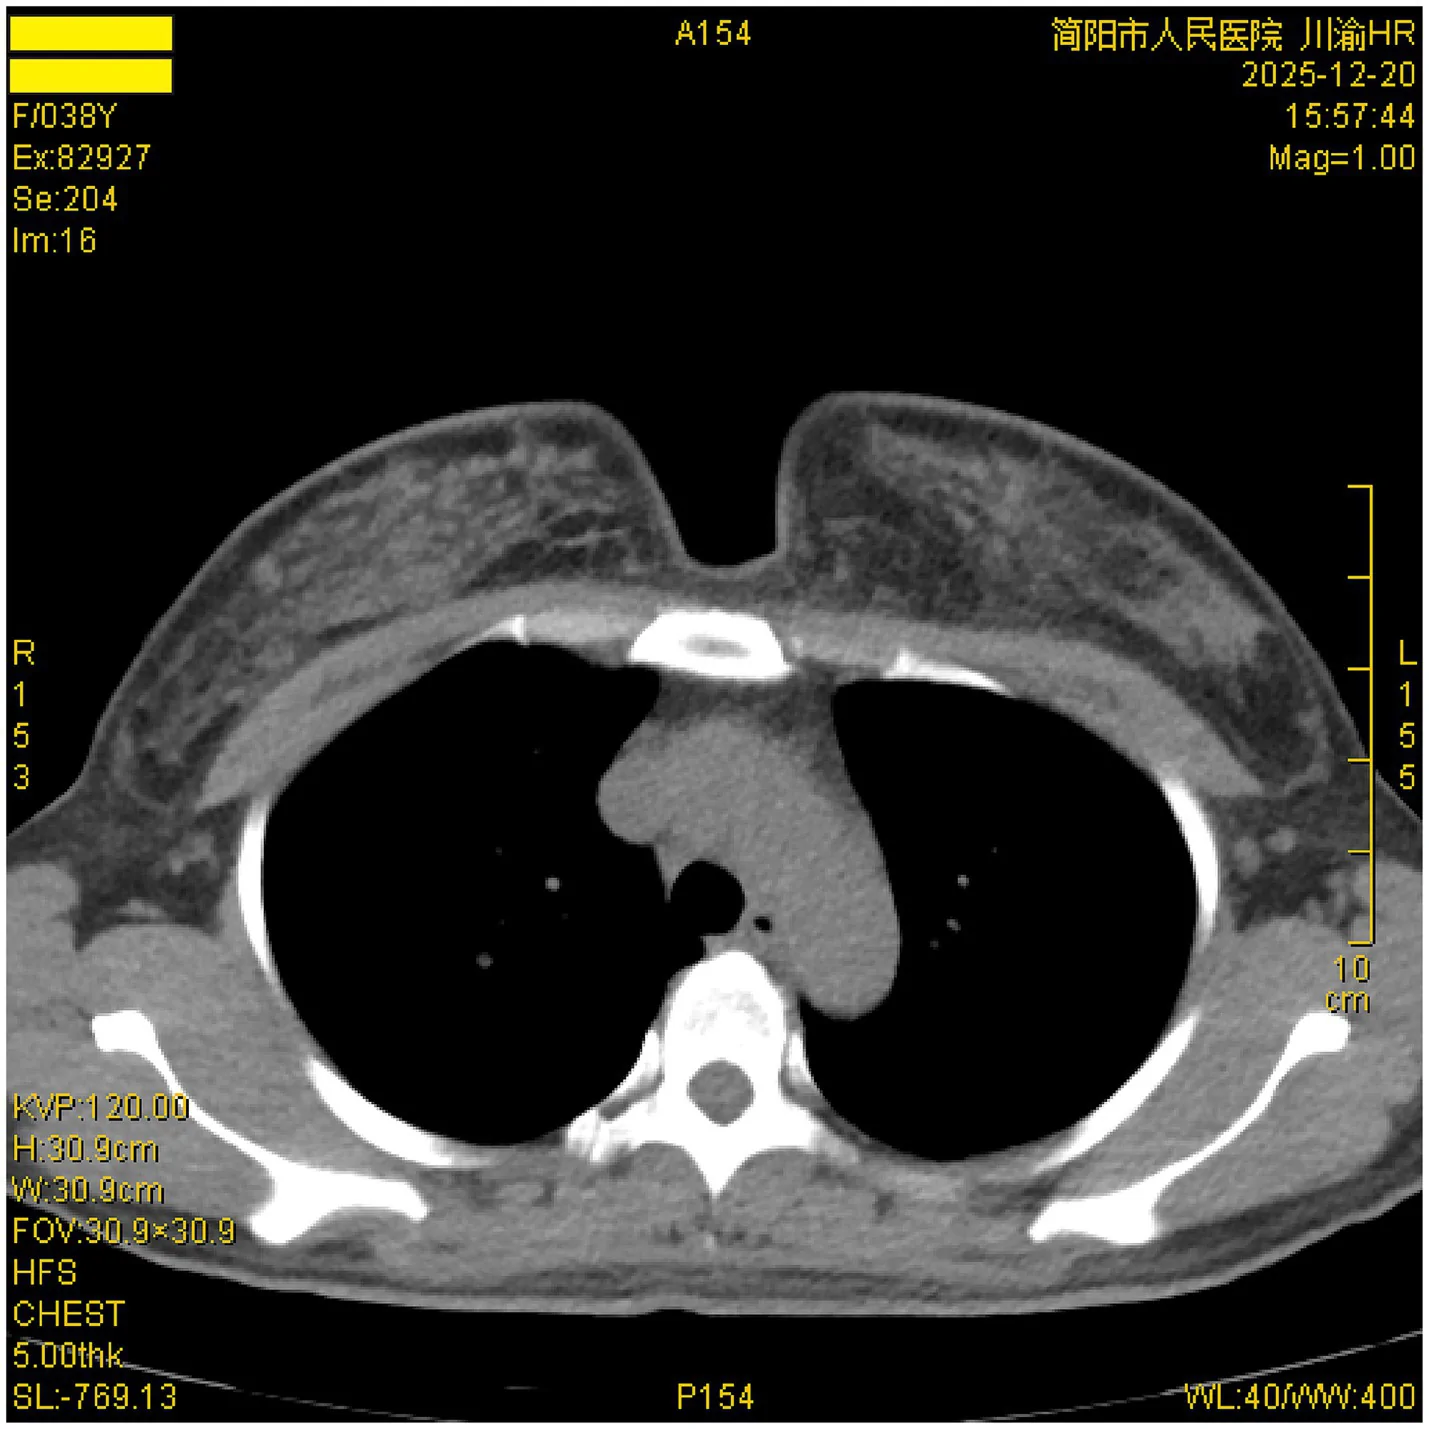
Chest computed tomography (CT) scan. CT image demonstrating pericardial effusion.
